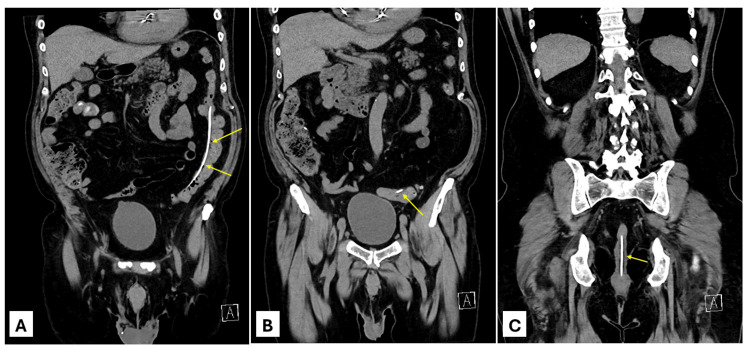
Coronal CT images at presentation to the emergency department. Yellow arrows indicate the distal catheter. (A) VPS tubing adjacent to the descending colon. (B) Shunt tubing perforating into the lumen of the descending colon. (C) Shunt tubing within the lumen of the rectum. Notably, there is no evidence of peritoneal free fluid or pneumoperitoneum. CT: computed tomography, VPS: ventriculoperitoneal shunt
